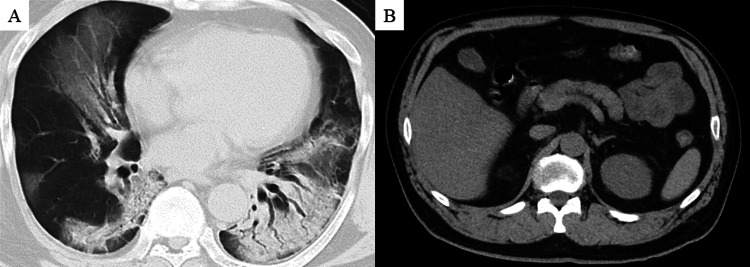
Chest and abdominal CT scans on admission (A) Chest CT scan shows bilateral ground-glass opacities and consolidation in both lungs. (B) Abdominal CT scan reveals normal gall bladder and biliary tract, with unremarkable pancreas CT: computed tomography
